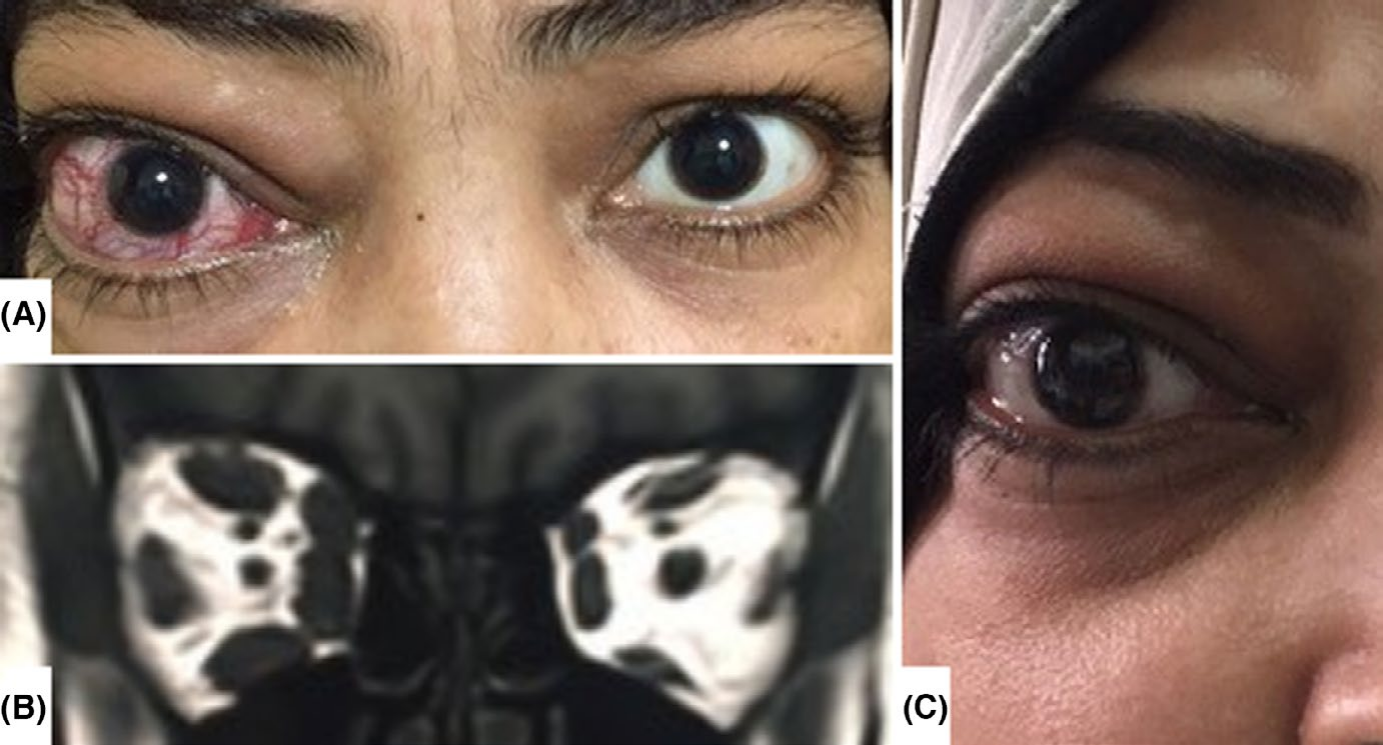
At presentation, there was redness, proptosis, upper eyelid edema and retraction, and increased inferior scleral show of the right eye compared with the left, with a clinical activity score (CAS) of 5/7 (A). Magnetic resonance imaging of the orbit revealed an enlargement of the extraocular muscles of the right eye with tendon sparing compared with the left (B). Six months following methotrexate monotherapy, there was marked improvement of the clinical condition apart from mild residual conjunctival chemosis (C)
